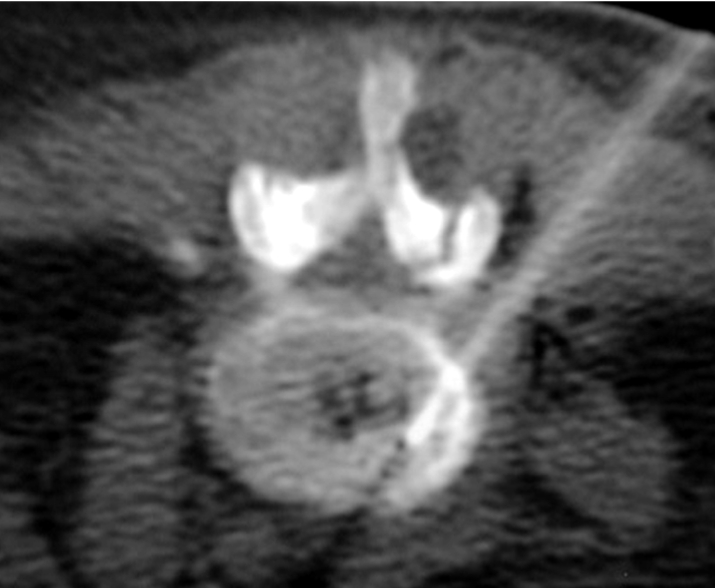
Biopsy of the L4/5 disc using the posterolateral paravertebral approach in a 66-year-old woman with infective discitis. CT fluoroscopic image taken with the patient lying prone shows the tip of the Ostycut needle within the right side of L4/5 disc. Note the streak artefact indicating the tip of the needle.
